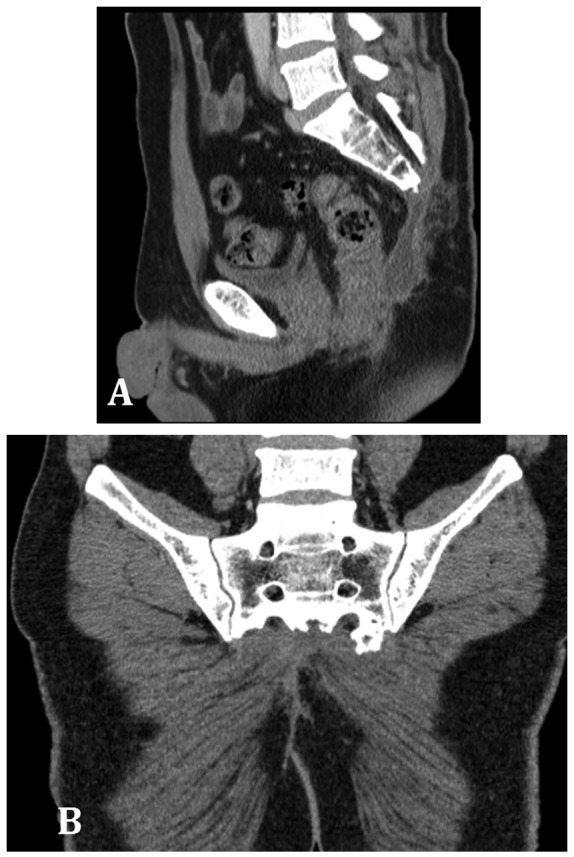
Case one. (A) Sagittal and (B) coronal plane computed tomography scans showing no local recurrence at the last follow-up examination 33 months after the first surgery.
